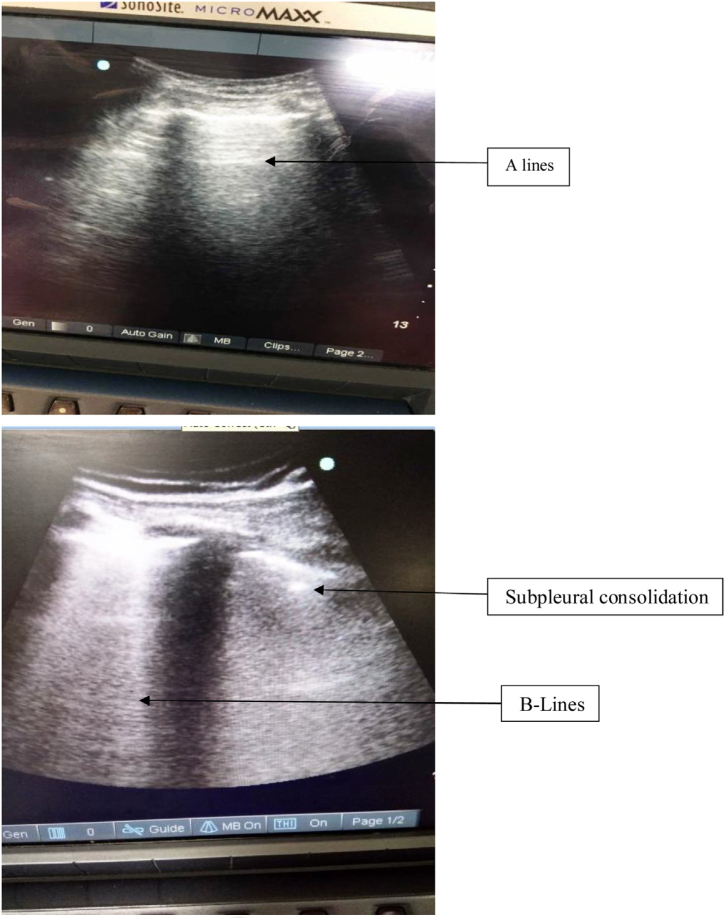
Lung ultrasonography of right upper and lower quadrants. Lower quadrant shows the presence of subpleural consolidation and multiple B lines.
